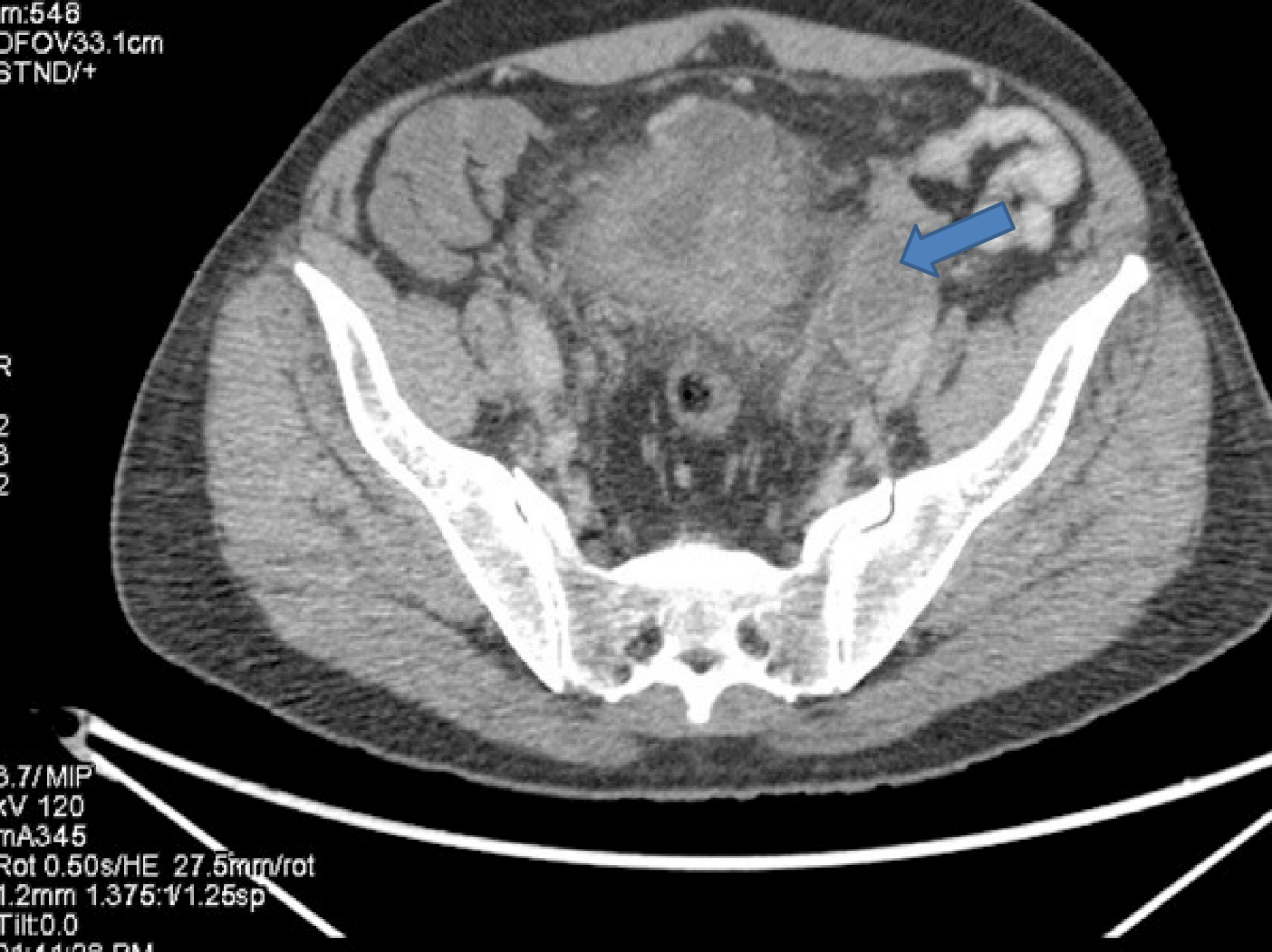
Computed tomography (CT) showing progression in left internal iliac lymph nodes.
